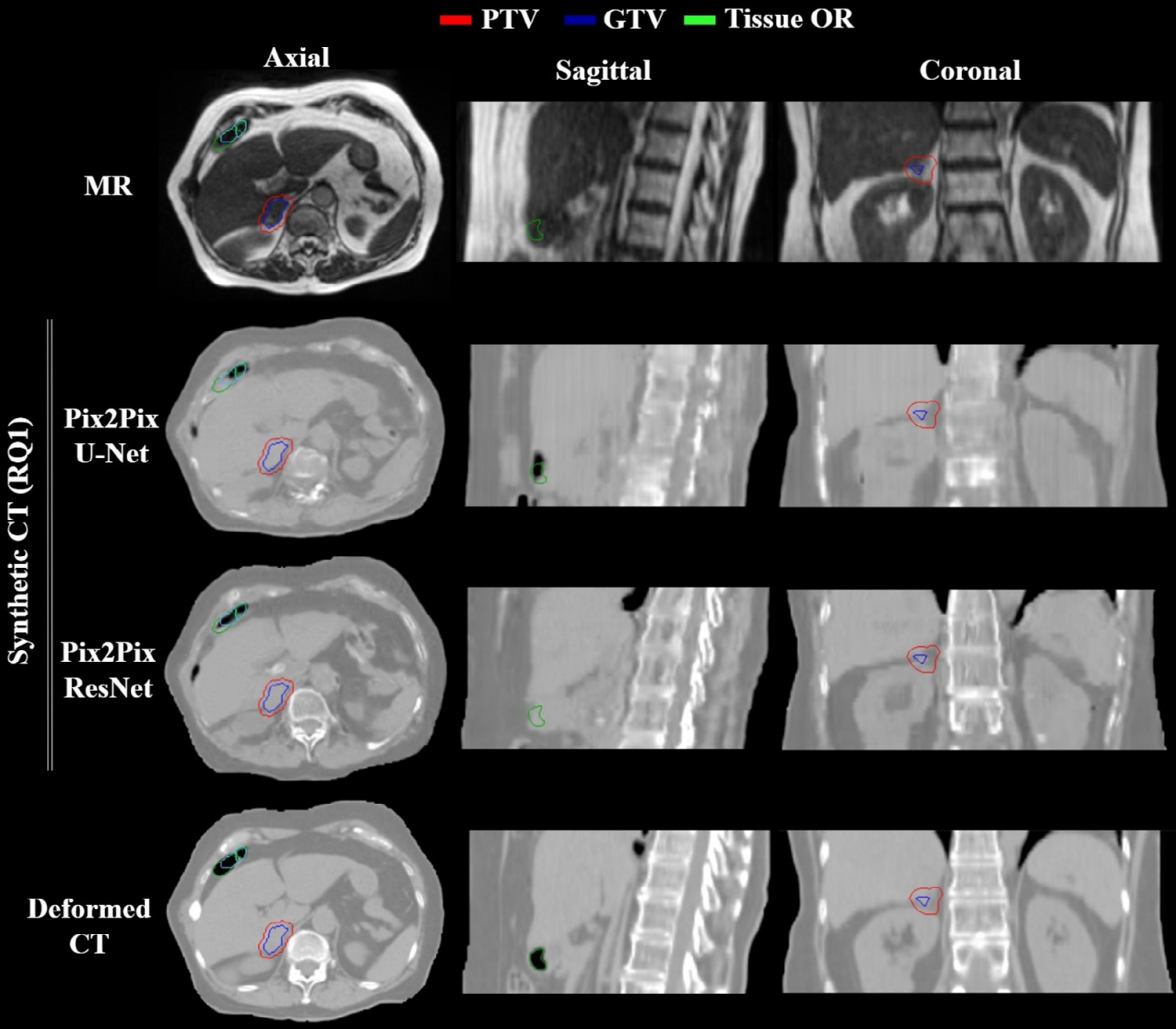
Research question 1. Example of sCT volumes generated with the help of U‐Net and ResNet generators for an adrenal gland case. The axial and sagittal slices reveal the ability of the ResNet to obtain sharper organ boundaries while outperforming U‐Net in the ability to avoid manual air and soft tissue bulk density override (Tissue OR) steps by automatically filling these structures correctly. The coronal slices show the performance in the bone region. The window width and window level of the shown CT and sCT images are 844 and ‐100 HU.
